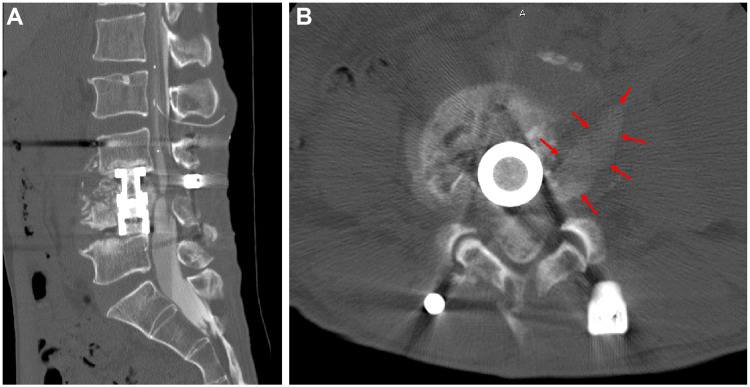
A postmyelography CT scan in sagittal (A) and axial (B) planes revealed leakage of contrast medium at the L4 level into the retroperitoneal space (arrows).
